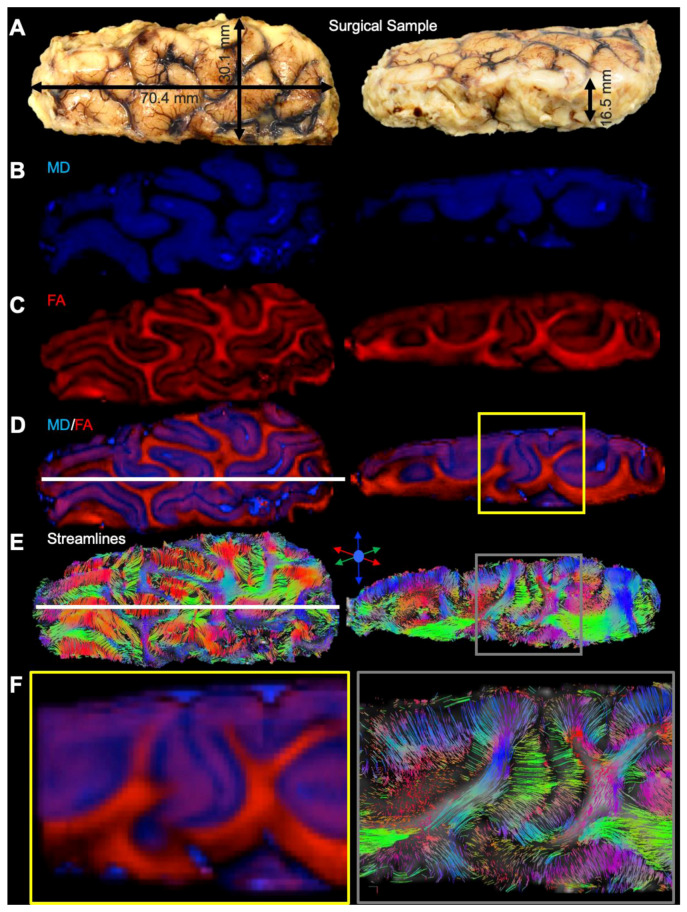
Diffusion tensor imaging (DTI) of surgically excised cortical dysplasia samples. (A) Surgically excised samples consisted of the middle temporal gyrus (MTG) and were measured in each dimension in transverse and sagittal plane to define image dimension required for MR imaging. (B) The surface identification of gyri was readily visualized in mean diffusion (MD) images, reflecting the high cellularity of the grey matter versus the lack of cellularity in sulci. (C) In contrast to MD images, high fractional anisotropy (FA) value were evident in white matter that passed in between gyri. (D) Color-coding of MD (blue) and FA (red) images afforded an overlay of both contrasts. A combined image provided a better definition of microenvironments that aided in the segmentation of regions of interest. (E) Tractography of these samples further revealed streamlines that connected grey matter through white matter and afforded a unique view of individual gyri. (F) A cut-out region of the samples highlights the exquisite detail that defines the cerebral mantle versus the underlying white matter, as well as individual streamlines that fan out from the white into grey matter in individual gyri.
